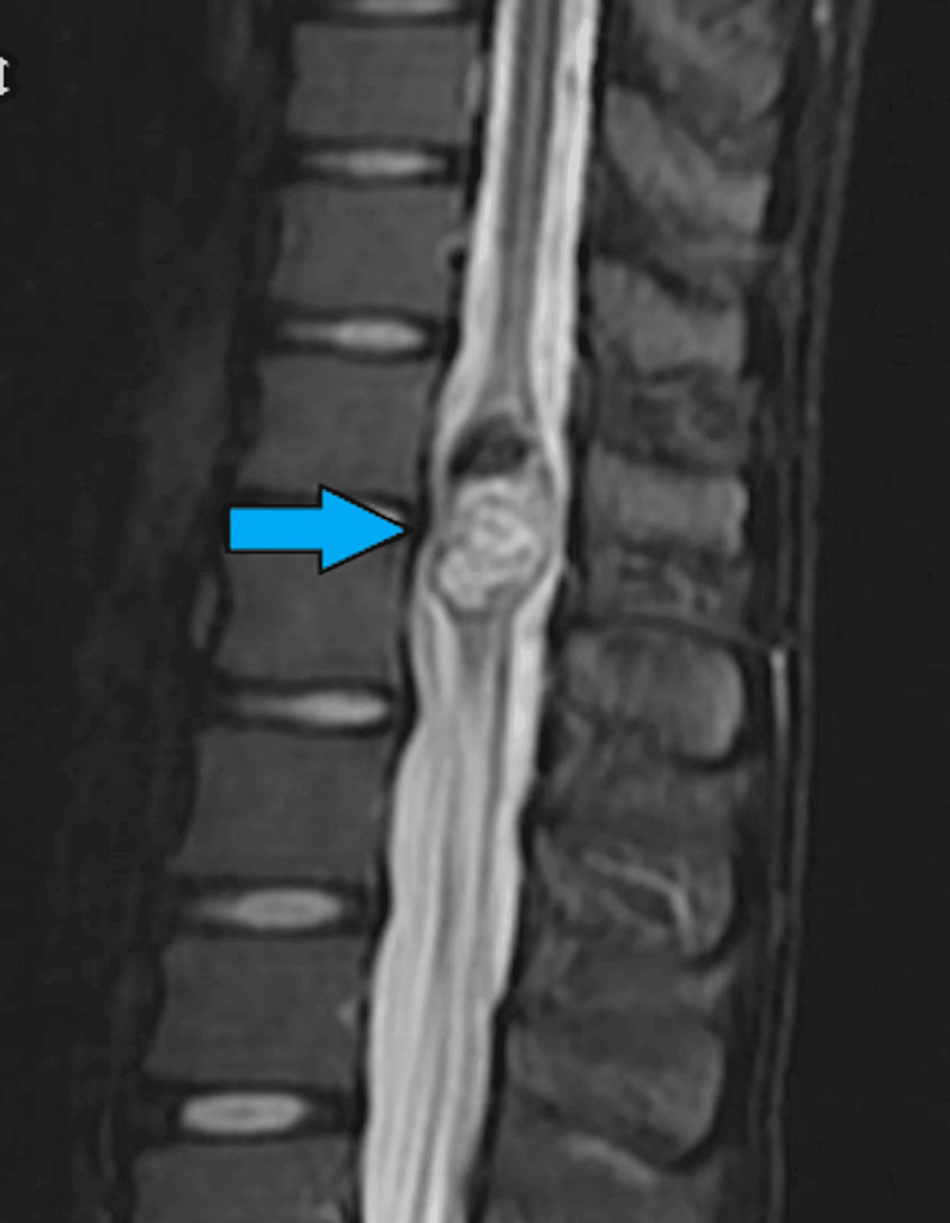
T2 fat-suppressed MRI depicting an intramedullary lesion within the spinal cord at D12-L1 level (size: 1.4 cm anteroposterior and 1.85 cm transverse; blue arrow).
